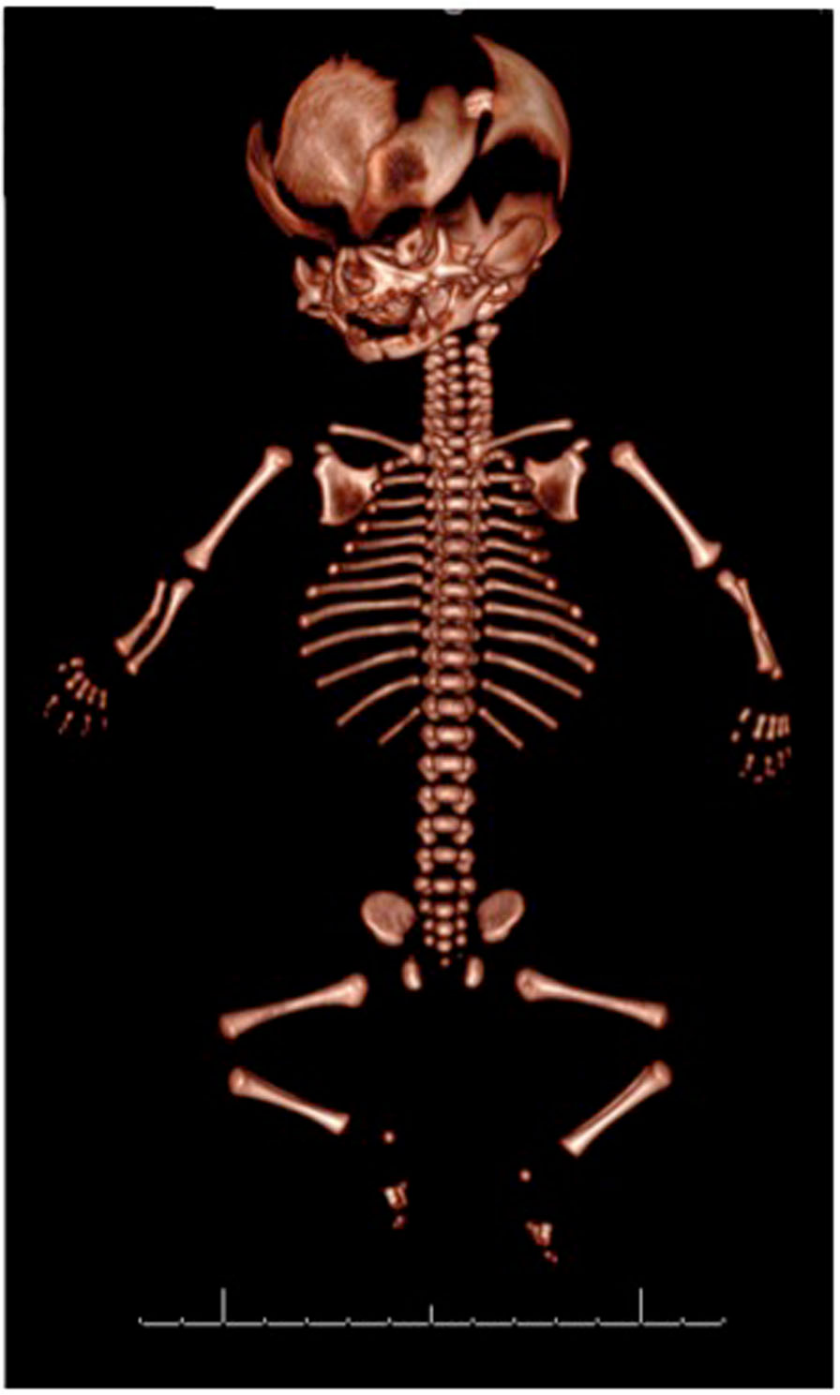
Post delivery CT images confirming short thin gracile bones and the abnormal skull with basal cranialstenosis
